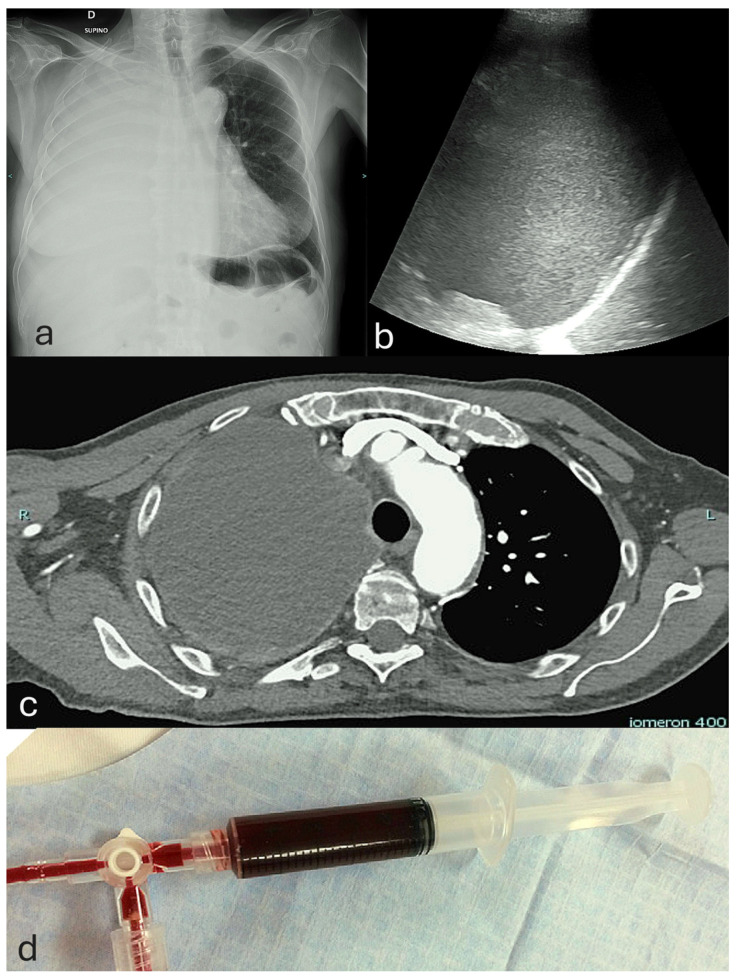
Multimodal imaging of hemothorax: (a) Chest X-ray (supine view) showing homogeneous opacification of the right hemithorax due to a large pleural effusion with contralateral mediastinal shift. (b) Thoracic ultrasound with a convex probe demonstrating a homogeneously echogenic pleural effusion suggestive of hemothorax in the acute or early subacute phase, reflecting the high cellularity of blood and resulting in marked acoustic reflection. (c) Contrast-enhanced chest computed tomography confirming the presence of hyperdense pleural fluid, consistent with hemothorax. (d) Pleural fluid aspiration yielding frankly hemorrhagic fluid. Hemothorax was definitively confirmed by a pleural fluid-to-blood hematocrit ratio ≥ 50% of the peripheral blood hematocrit.
